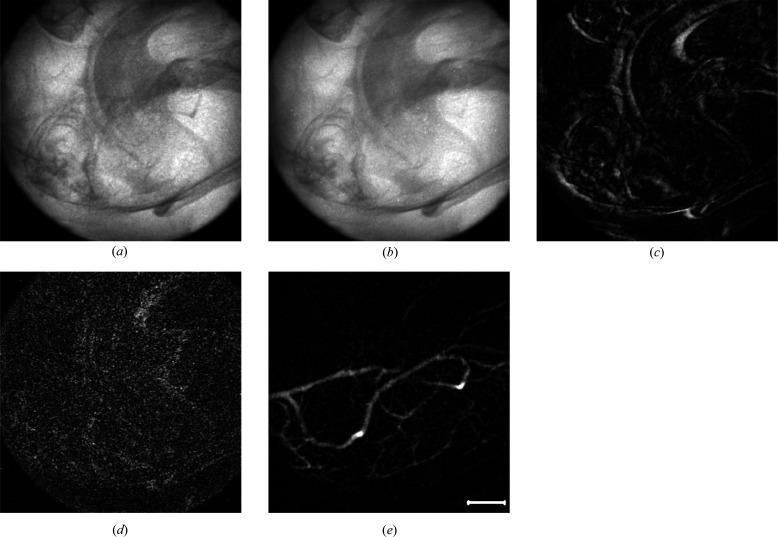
The experimental results for MCXI with a X-ray tube, where (*a*) is a single image frame of the raw angiogram, (*b*) is the time-averaged image of the raw angiogram; (*c*) is the image of moving tissues reconstructed by MCXI; (*d*) represents the high-frequency noises reconstructed by MCXI and (*e*) represents the blood vessels delineated by MCXI. The scale bar represents 2000 µm.
